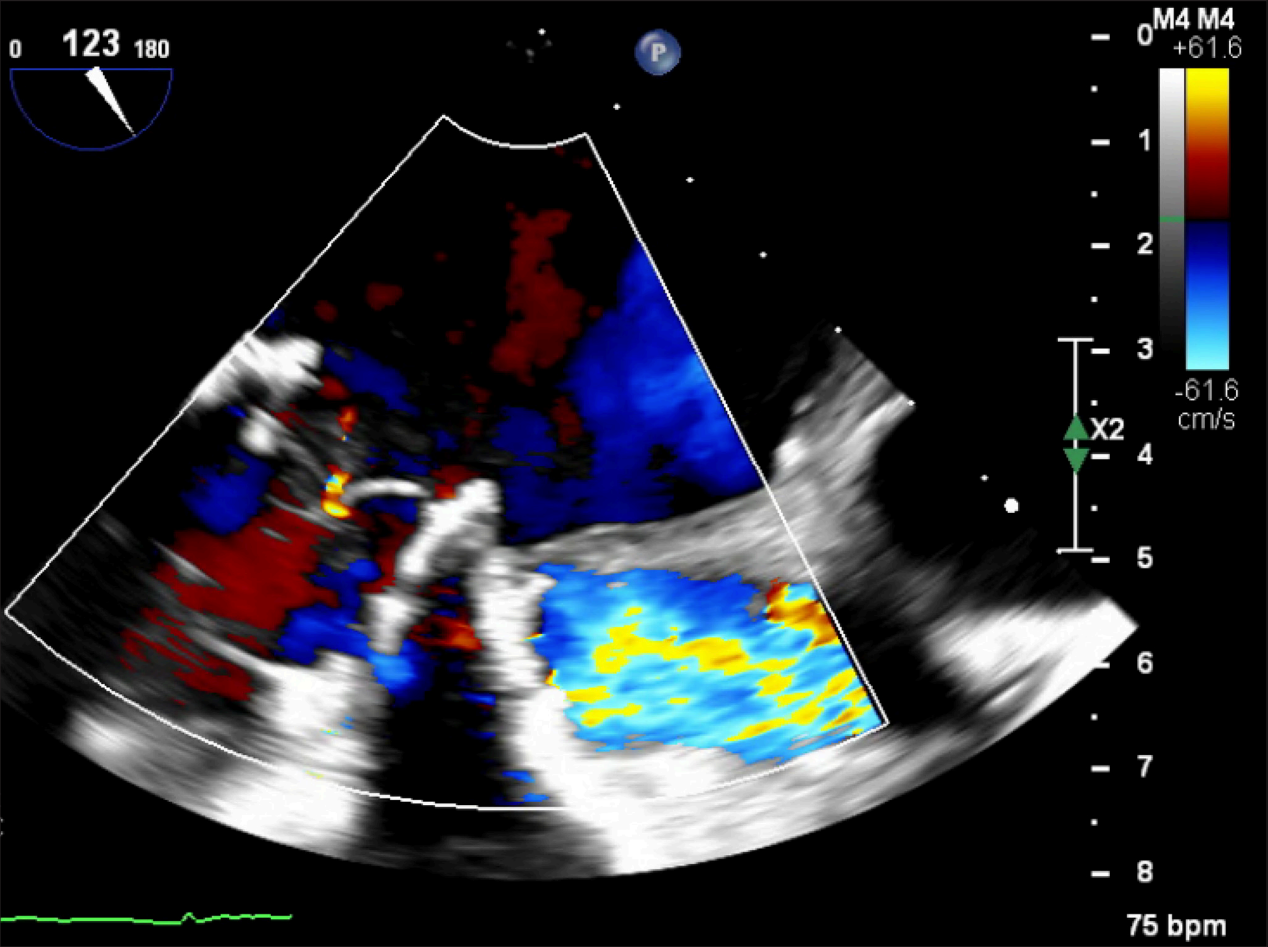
Transesophageal echocardiogram showing trivial mitral regurgitation after valve-in-valve procedure.
